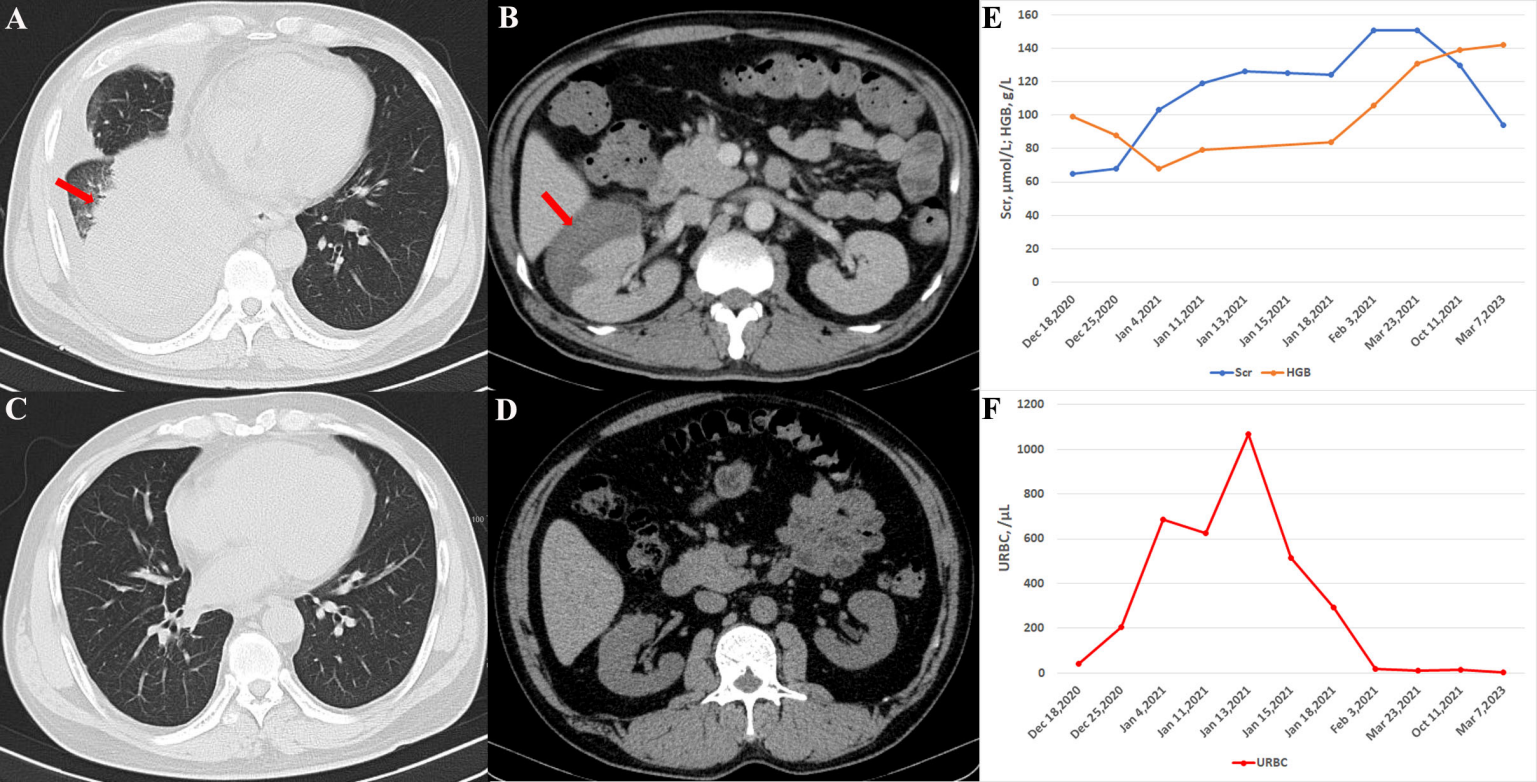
Imaging and laboratory testing of the patient. (A) Chest CT showing pulmonary consolidation (arrow) in the right lower lung upon admission. (B) The abdominal CT scan displays a large right-sided perirenal hematoma (arrow). (C) Six months later, the chest CT indicated complete resolution of right lung consolidation. (D) Perirenal hematoma disappeared six months after treatment. (E) Hemoglobin levels dropped to 68 g/L and serum creatinine rose to 103 *µ*mol/L on January 4, 2021, although both parameters gradually normalized following treatment. (F) Urine red blood cell count, which had significantly increased on January 4, 2021, also returned to normal after treatment.
